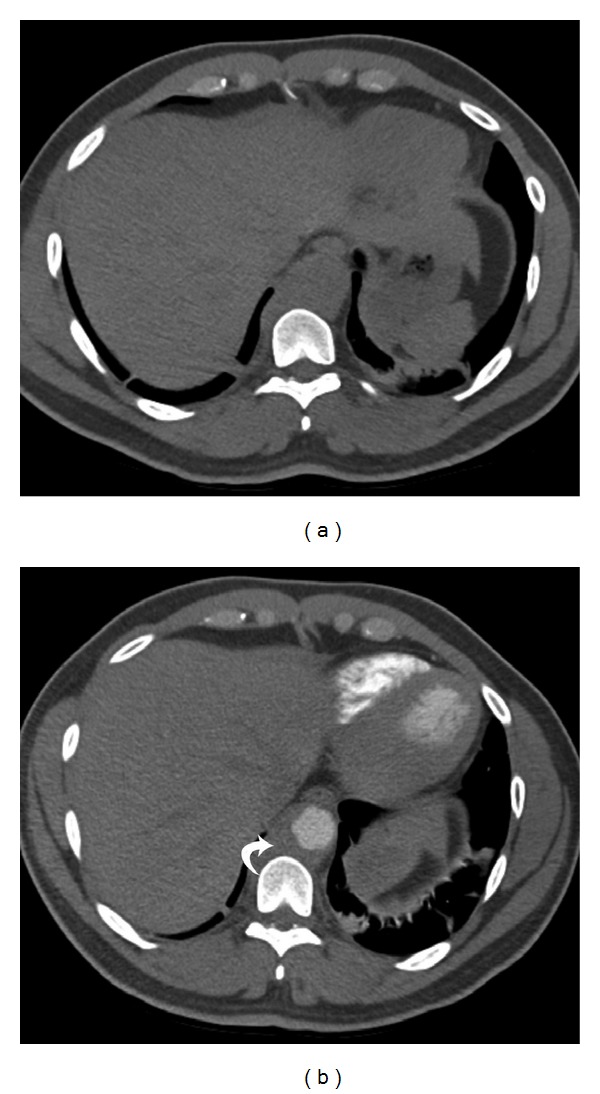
Contained rupture—(a) noncontrast CT scan in a patient presenting with acute chest pain shows nonspecific soft tissue adjacent to descending thoracic aorta. (b) Contrast enhanced CT at the same level shows lack of distinction between the posterior wall of the aorta and the adjacent vertebral body, the “draped aorta” sign (curved arrow), which is indicative of a contained rupture.
